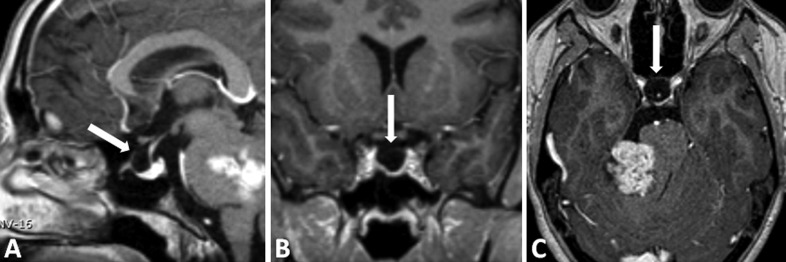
Preoperative magnetic resonance imaging (MRI) (A) Sagittal, (B) coronal, and (C) axial T1-weighted gadolinium-enhanced MRI in the same patient revealed an enlarged sella relative to a normal pituitary gland.
